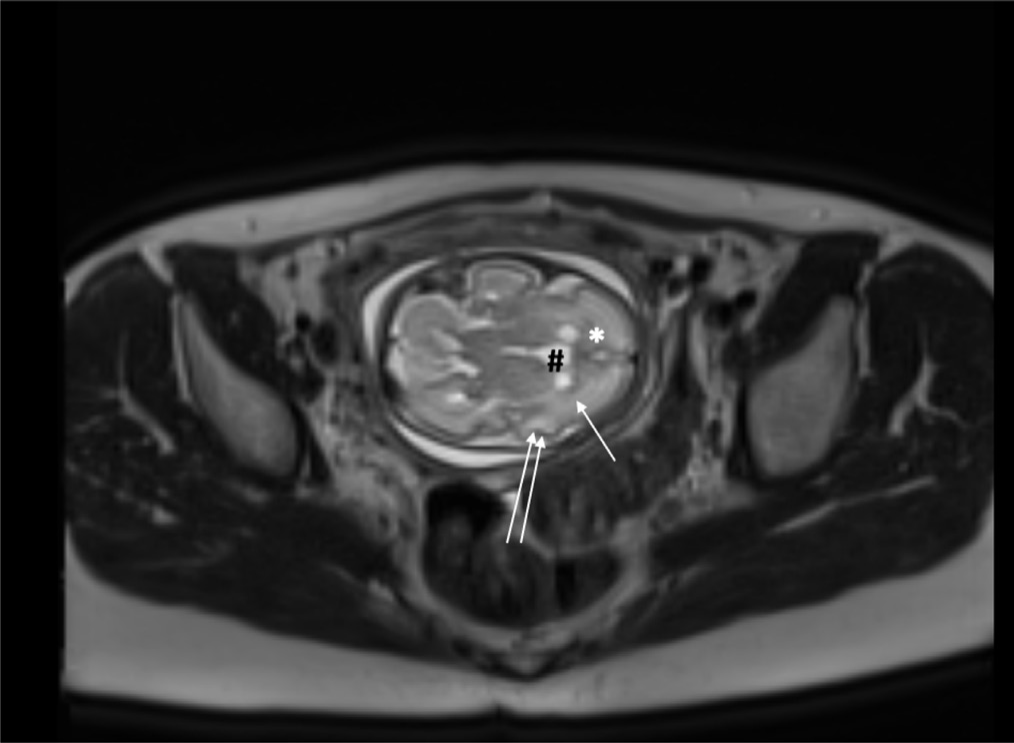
T2 haste axial section fetal MRI, the fetal brain shows abnormal changes (increased differentiation between the white (arrow) and grey matter (double arrow), bulky cavum septum pellucidum (#) and prominent neuronal migration pattern, seen as bands in the white matter of the frontal lobe (*)).
